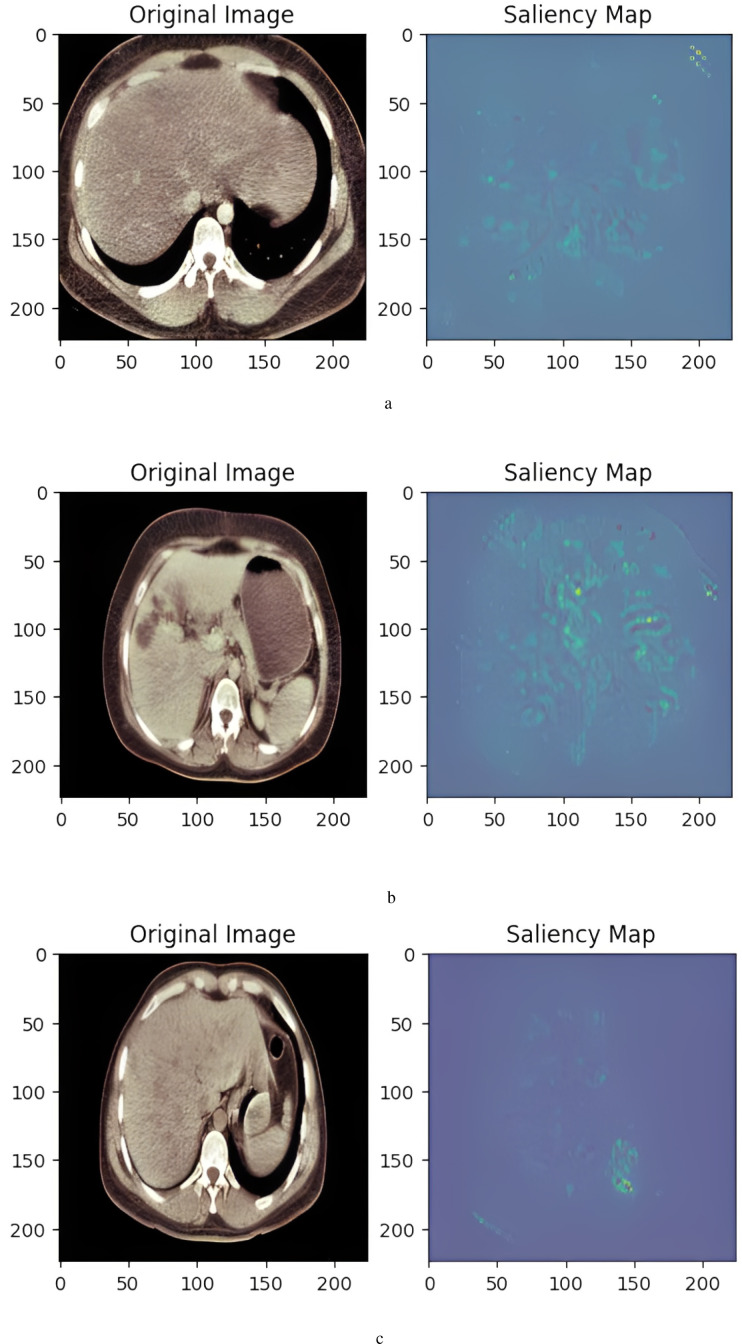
Saliency map interpretations of CT liver tumor images. Pixel-wise sensitivity maps for (a) Normal, (b) Benign, and (c) Malignant classes, emphasizing regions critical to model decisions.
